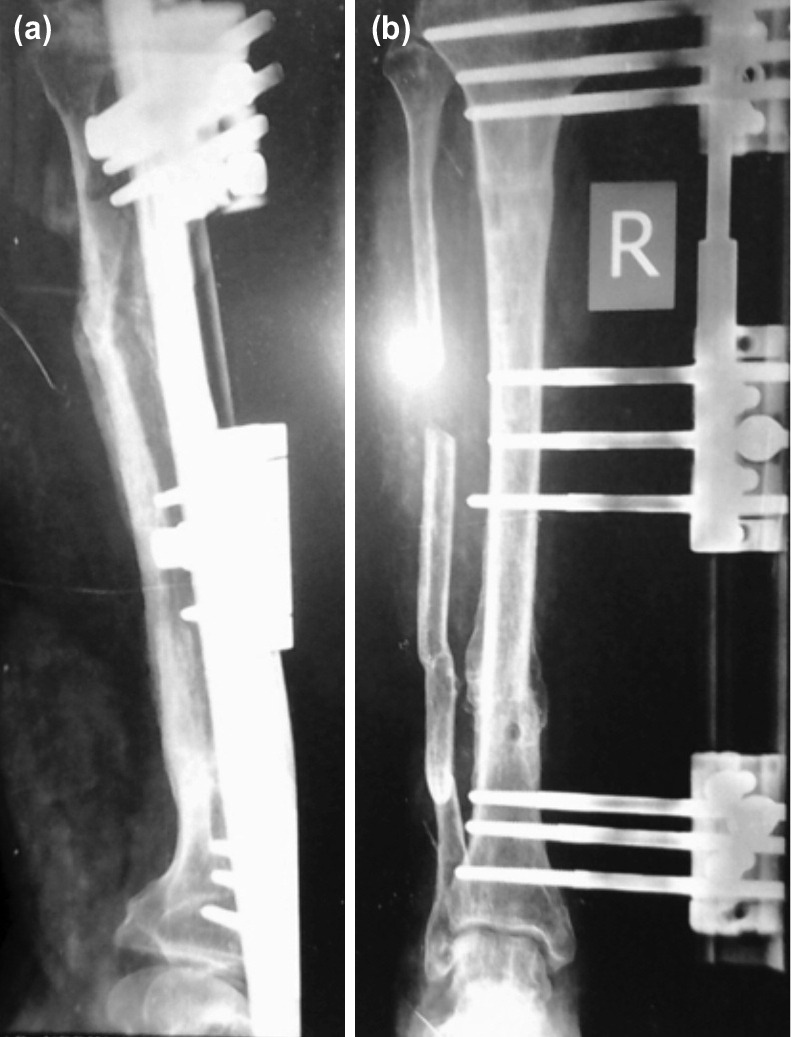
(a) Anterior posterior and (b) lateral plain radiograph showing Union achieved.
